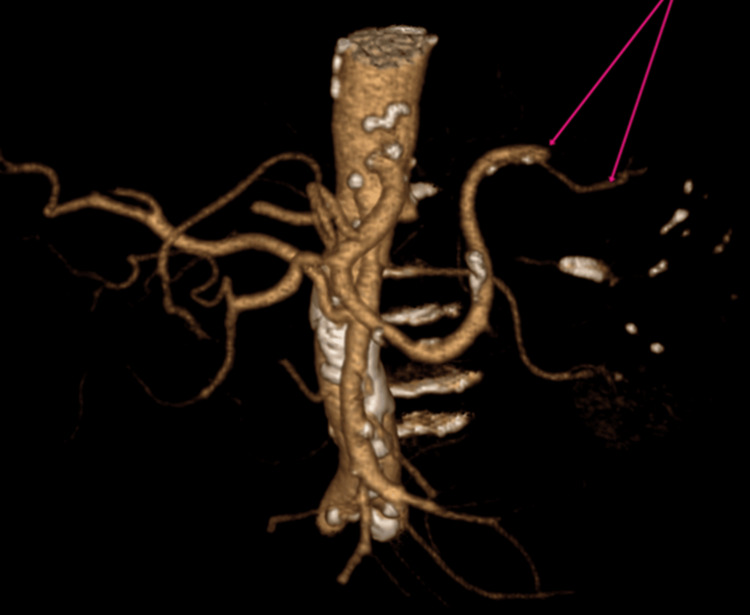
3D-volume-rendered CT angiography demonstrating abrupt cessation of contrast opacification in the distal splenic artery (pink arrows), indicating complete thrombosis. Proximal branches remain patent while distal perfusion is absent, correlating with extensive splenic infarction (Case 1)
